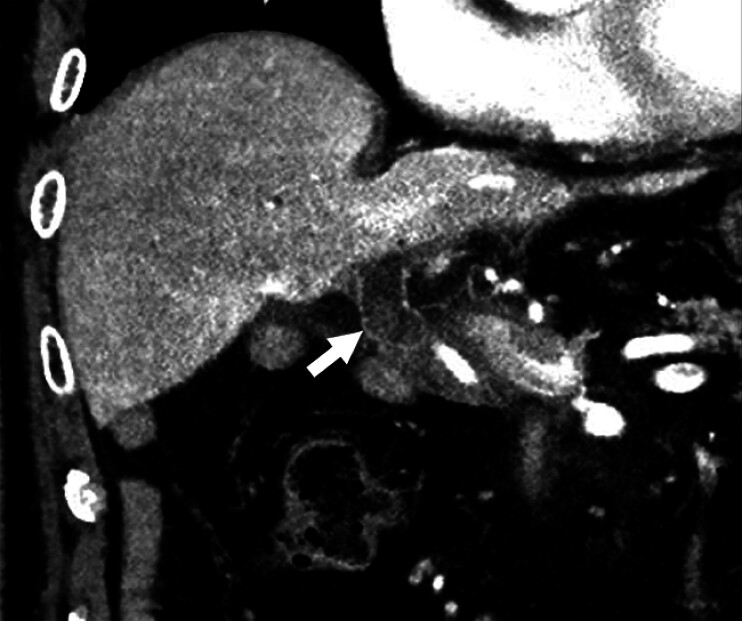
Computed tomography revealed an intrahepatic biliary stone in the coronal view (arrowhead).
